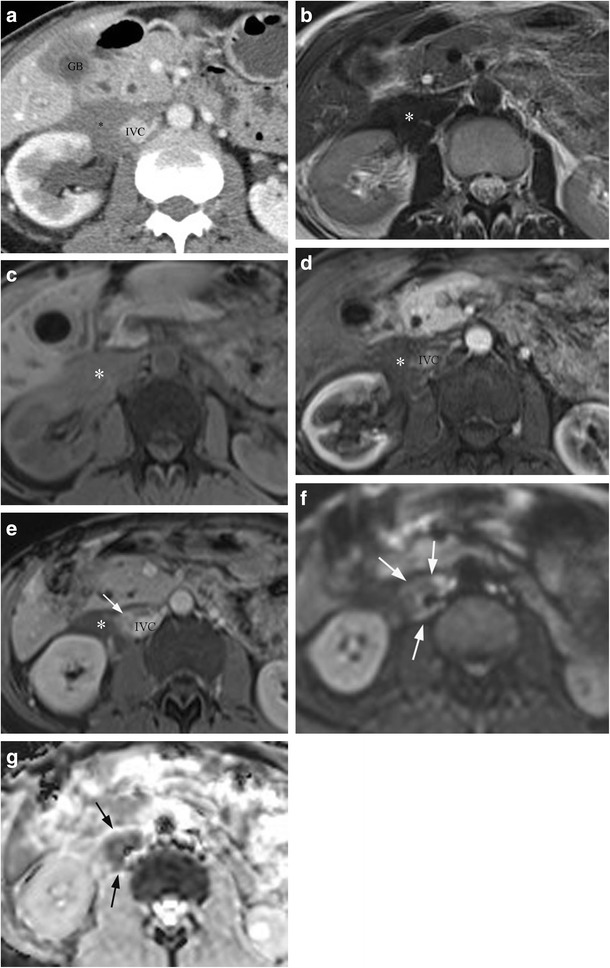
Example of extrarenal IgG4 disease. a CECT showing a rind of abnormal soft tissue (*) anterior to the right kidney and encasing the IVC. b) Axial T2 turbo spin echo image of the same patient as in Fig. 9a shows perirenal soft tissue is of low T2 signal (*). c Axial T1FS pre-contrast shows perirenal soft tissue of intermediate T1 signal (*). d Axial T1FS post-contrast arterial phase shows minimal enhancement of the perirenal soft tissue (*). e Axial T1FS post-contrast equilibrium phase shows delayed enhancement related to a cuff of soft tissue around the IVC (*arrow*) with most of the perirenal soft tissue remaining low T1 signal (*). f The enhancing tissue around the IVC shows restricted diffusion with high signal on DWI—B800 (*arrows*). g There is corresponding low signal on the ADC map (*arrows*)
